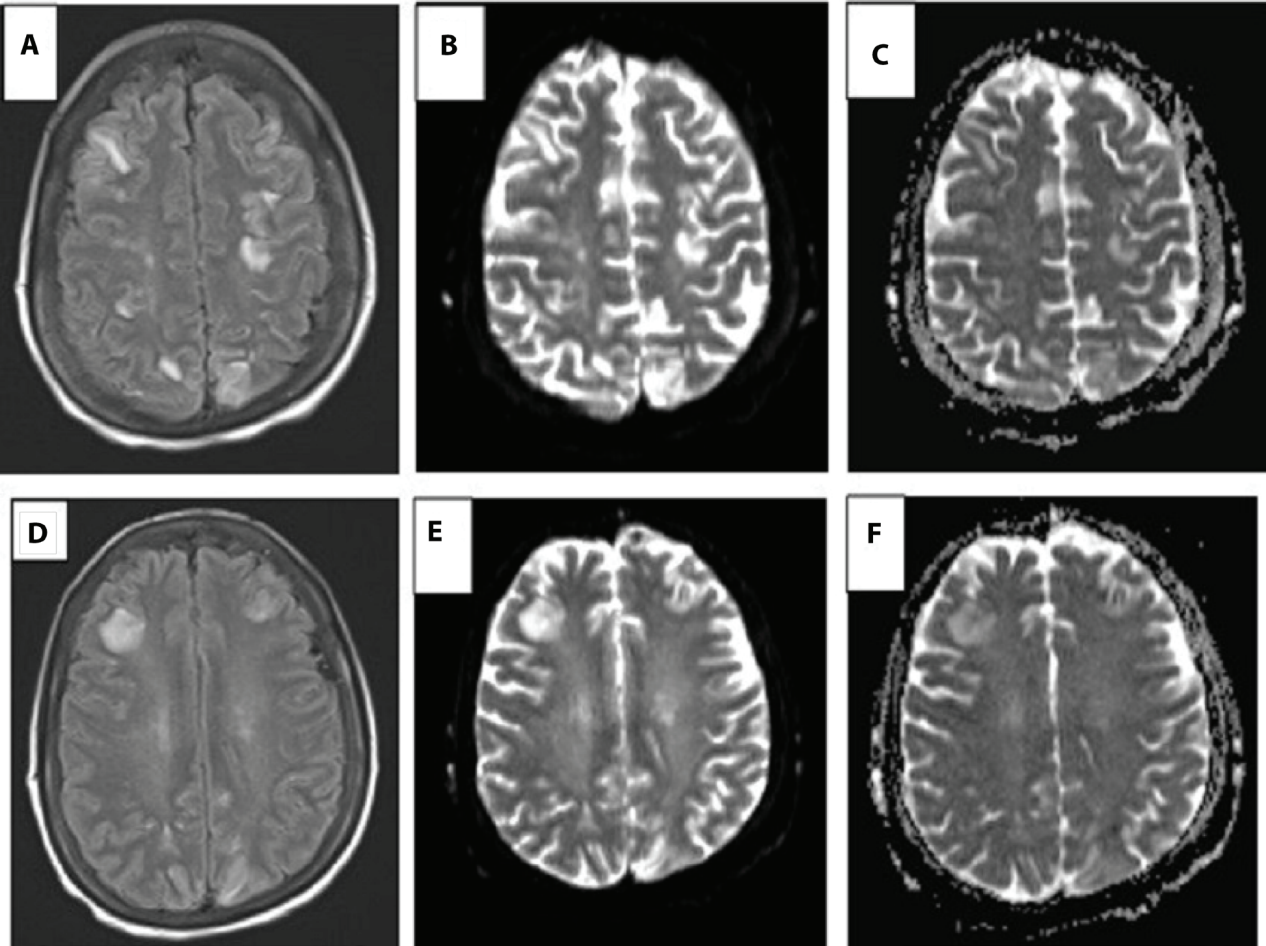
Brain MRI images consistent with PRES or posterior reversible leukoencephalopathy syndrome after lenvatinib treatment (A–C) demonstrate diffuse hyperintensities in the frontal and parieto-occipital cortices. (D–F) demonstrate localized edema around the right frontal lesion. (A, D) Fluid-attenuated inversion recovery imaging sequence (FLAIR). (B, E) Diffusion weighted imaging (DWI). (C, F) Apparent diffusion coefficient imaging (ADC)
